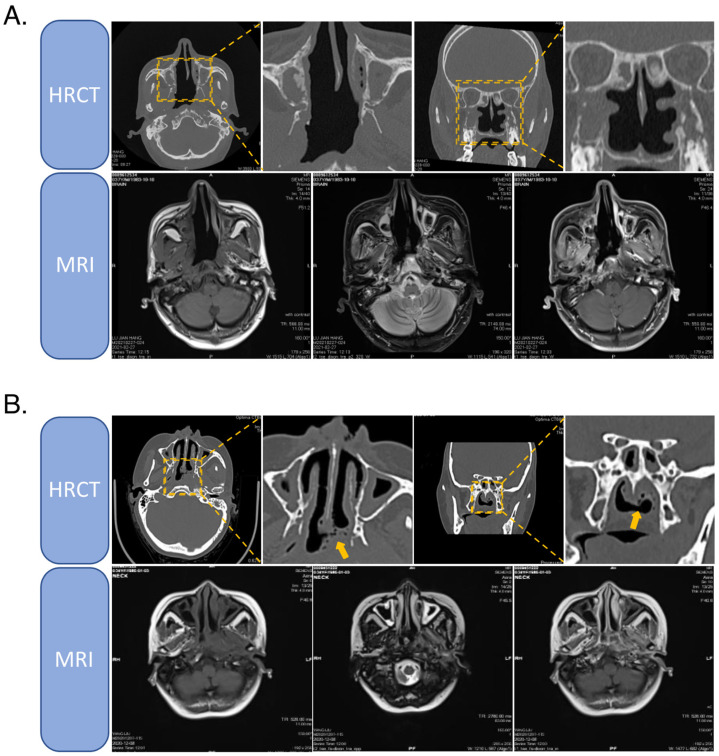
(A) HRCT and MRI images of bone necrosis in recurrent NPC patients. (B) HRCT and MRI images of soft tissue necrosis in recurrent NPC patients. An “air bubble shadow” can be seen in the soft tissue shadow on CT.
